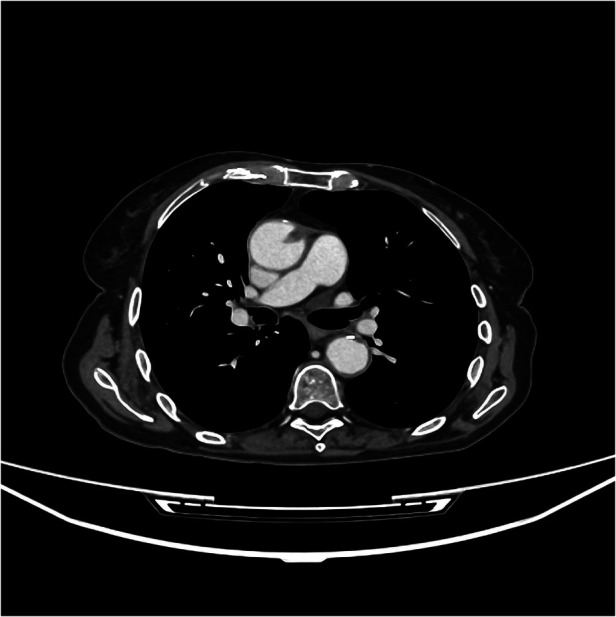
Transversal CT-scan plane showing the 8 mm thrombus in the ascending aorta.
